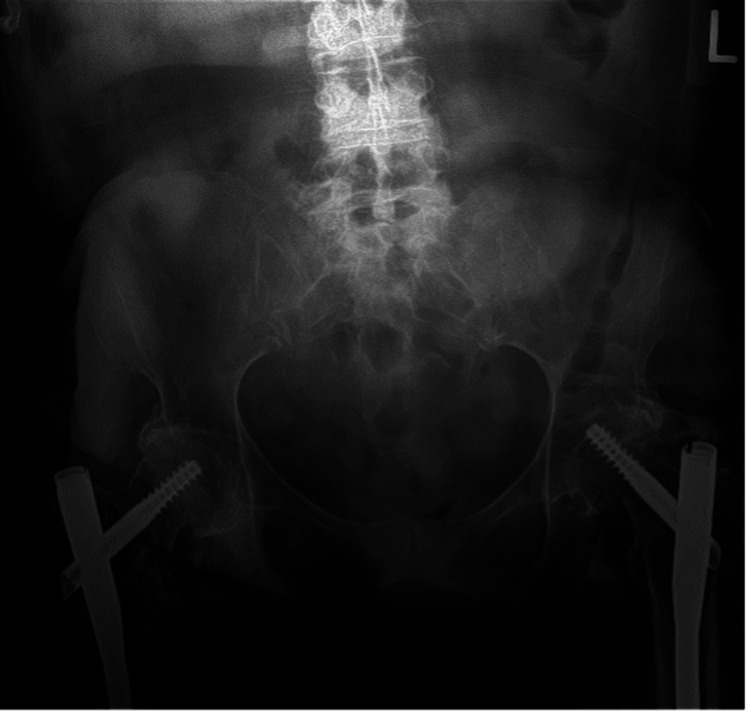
Six-week postoperative anteroposterior radiograph of the pelvis demonstrating mild collapse of the left proximal fracture and subsequent 1 mm of screw cut-out
